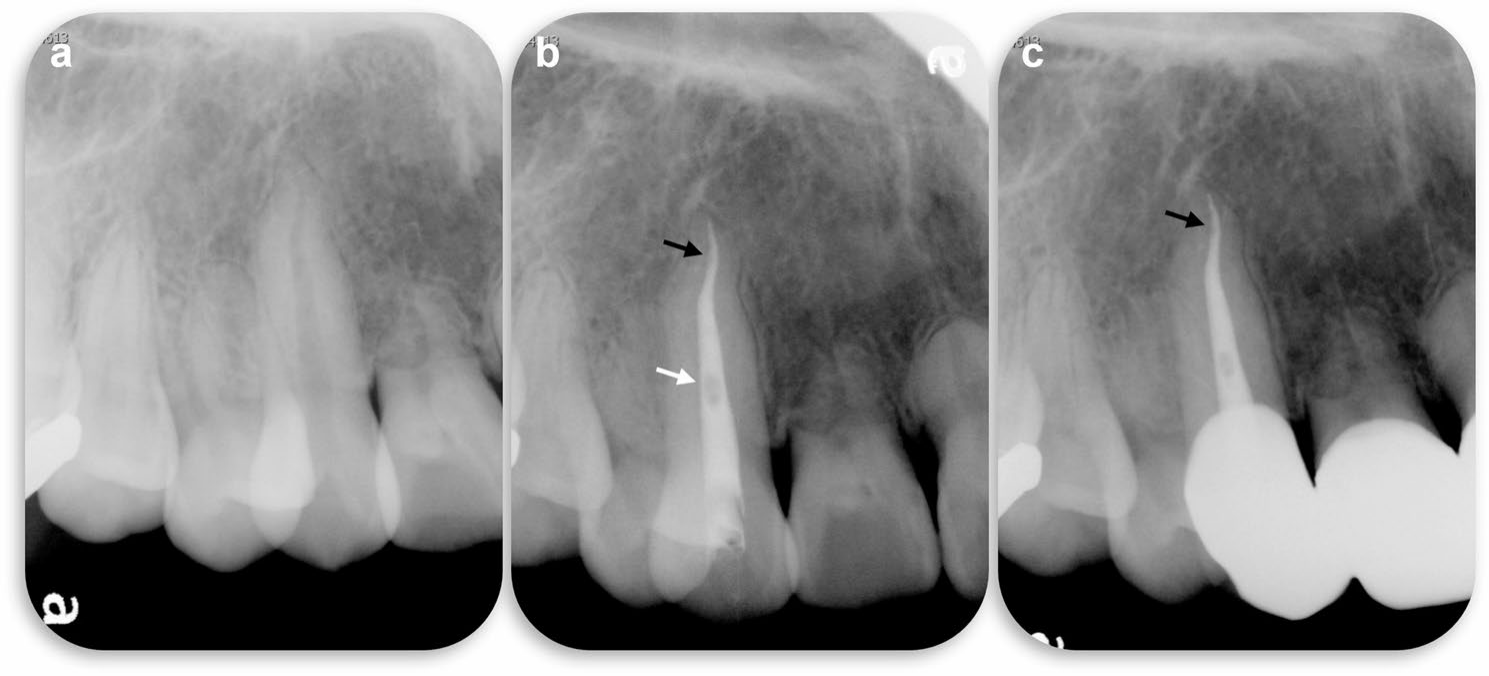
Case 2: Limitations and improvements of methacrylic resin-based root canal sealers without gutta-percha. (a) Pre-operative radiograph; (b) post-operative radiograph obtained immediately after RCF performed without gutta-percha showing a notable improvement with this obturation technique with the presence of only a small void (black arrow), while larger voids in the center of the canal (white arrow) may improve as prosthodontist gain more experience with techniques that do not rely on gutta-percha; (c) follow-up radiograph obtained 1 year after treatment showing no loss of sealer (black arrow)
